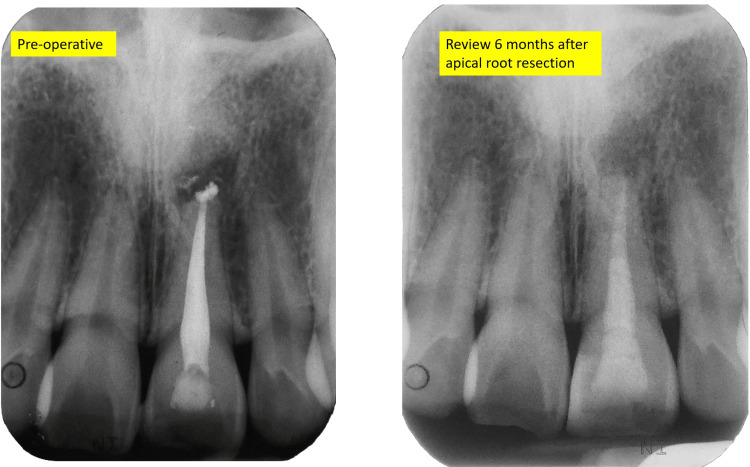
Periapical radiograph during six months of the review showing complete healing
